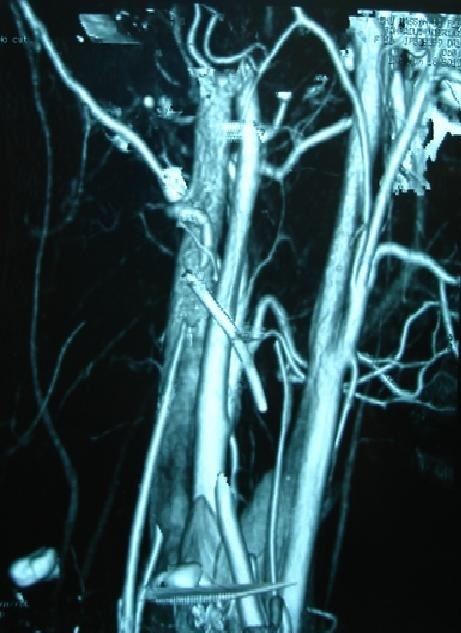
Cervical CT angiography that shows a metallic pin with interruption of blood flow within the left vertebral artery
